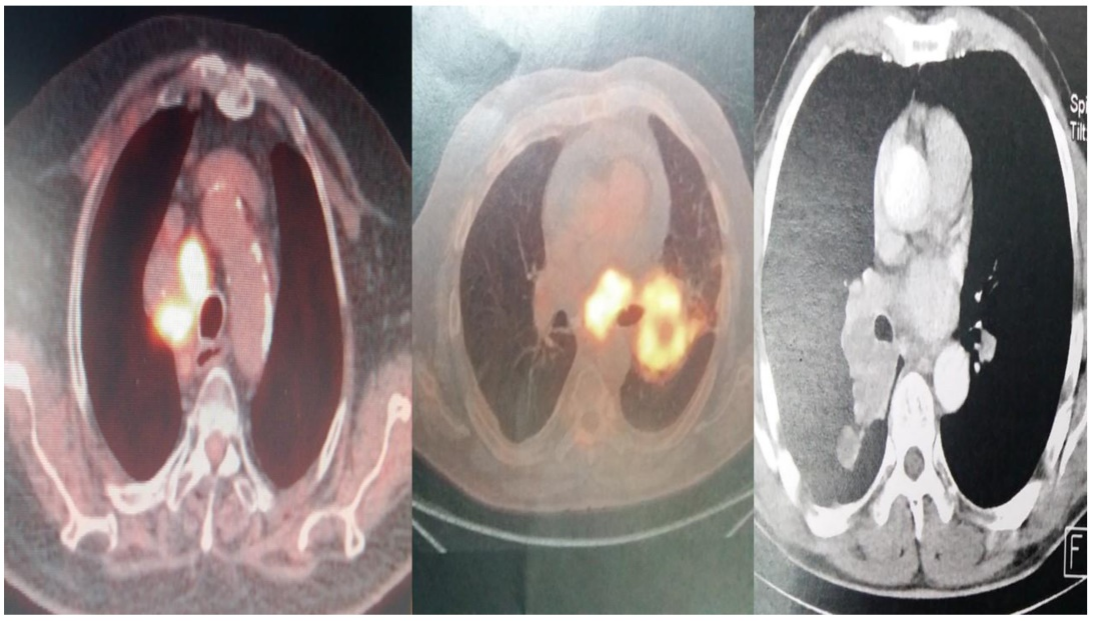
PET-CT and CT scan of different patients included
